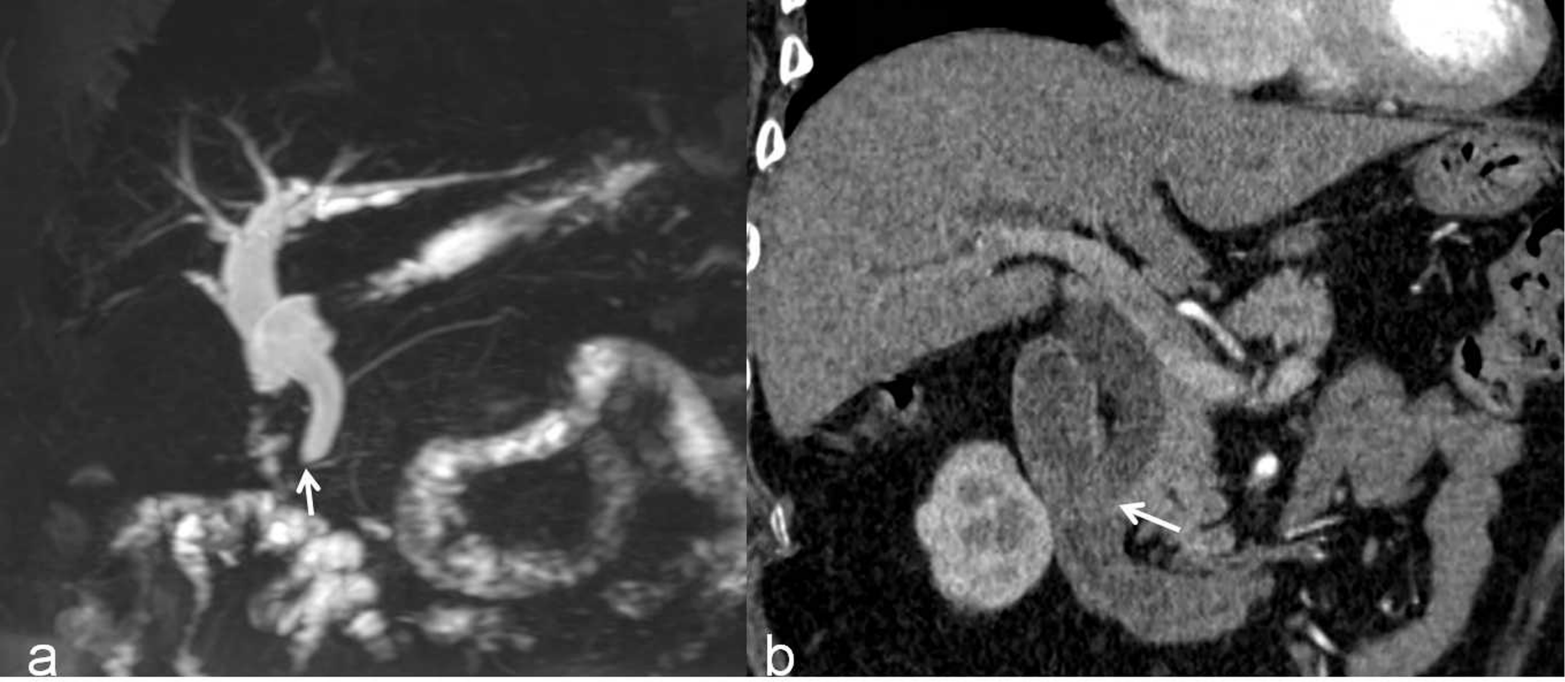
A benign DBS (score=0) in a 67-year-old woman who had a history of cholecystectomy. She did not require treatment but received imaging follow-up. (A) A 3D MRCP image shows a DBS with upstream bile duct dilatation. The stricture length is 4 mm, the angle of the DBS is 67.7°, and the double duct sign is not present. (B) A coronal CT MPR image demonstrates the isodensity of the wall in the arterial phase relative to the liver parenchyma (white arrow).
